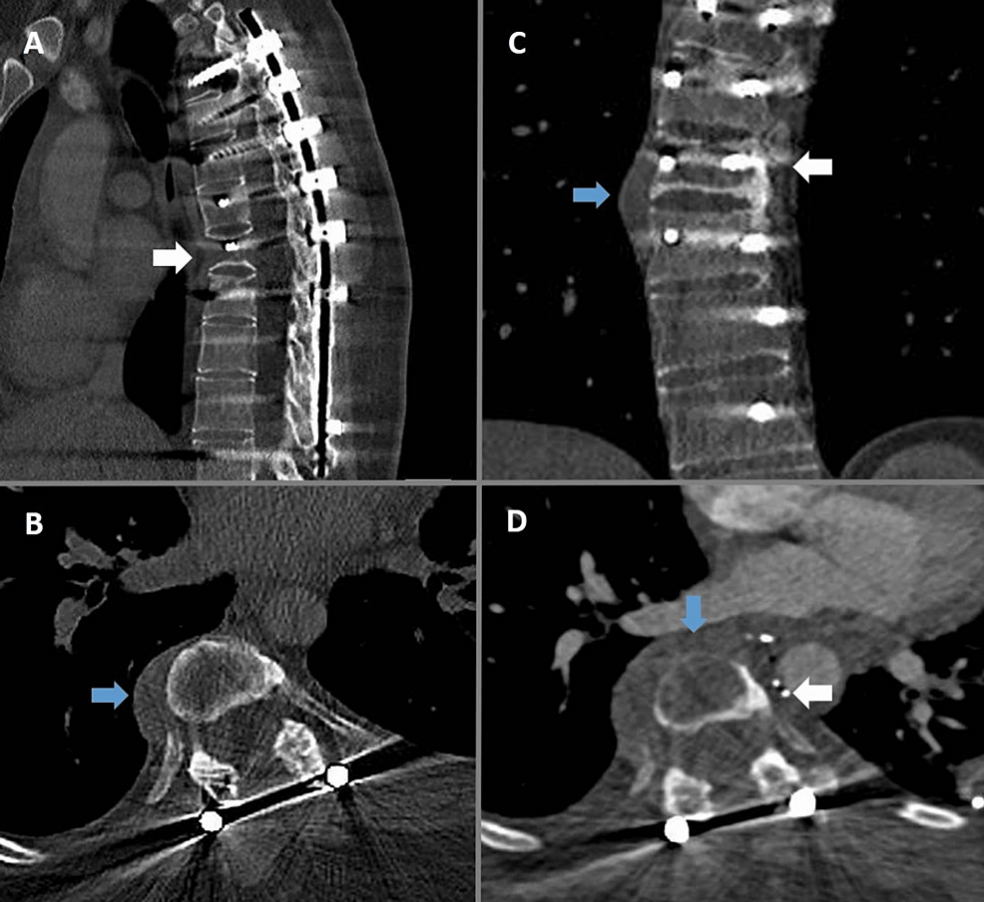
Case four Twenty-six-year-old female with T8 Osteosarcoma. All images are plain CT scans (A-C show pre-op imaging). Image A is a sagittal view demonstrating the former construct. Image B demonstrates an axial view through T8. Image C is a coronal view. Extraosseous tumor mass is indicated by the blue arrows and T8 Level by the white arrows. Image D is a post-thoracotomy axial image demonstrating an increased tumor anterior to T8 (blue arrow). Some surgical clips can be seen between T8 and the aorta (white arrow).
